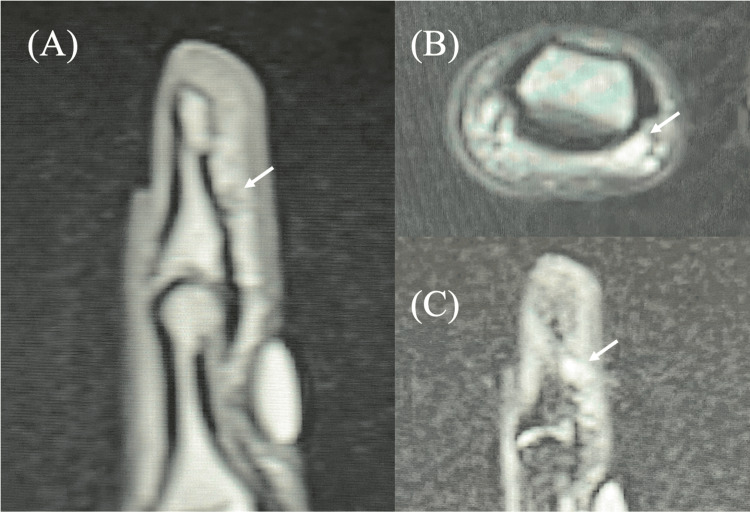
Preoperative MRI findings for case 4. A small nodular lesion is visible on the fingertip. (A) Low signal on a sagittal T1-weighted image. (B) Isointense signal on an axial T2-weighted image. (C) High signal intensity on a sagittal STIR image. STIR: Short tau inversion recovery
